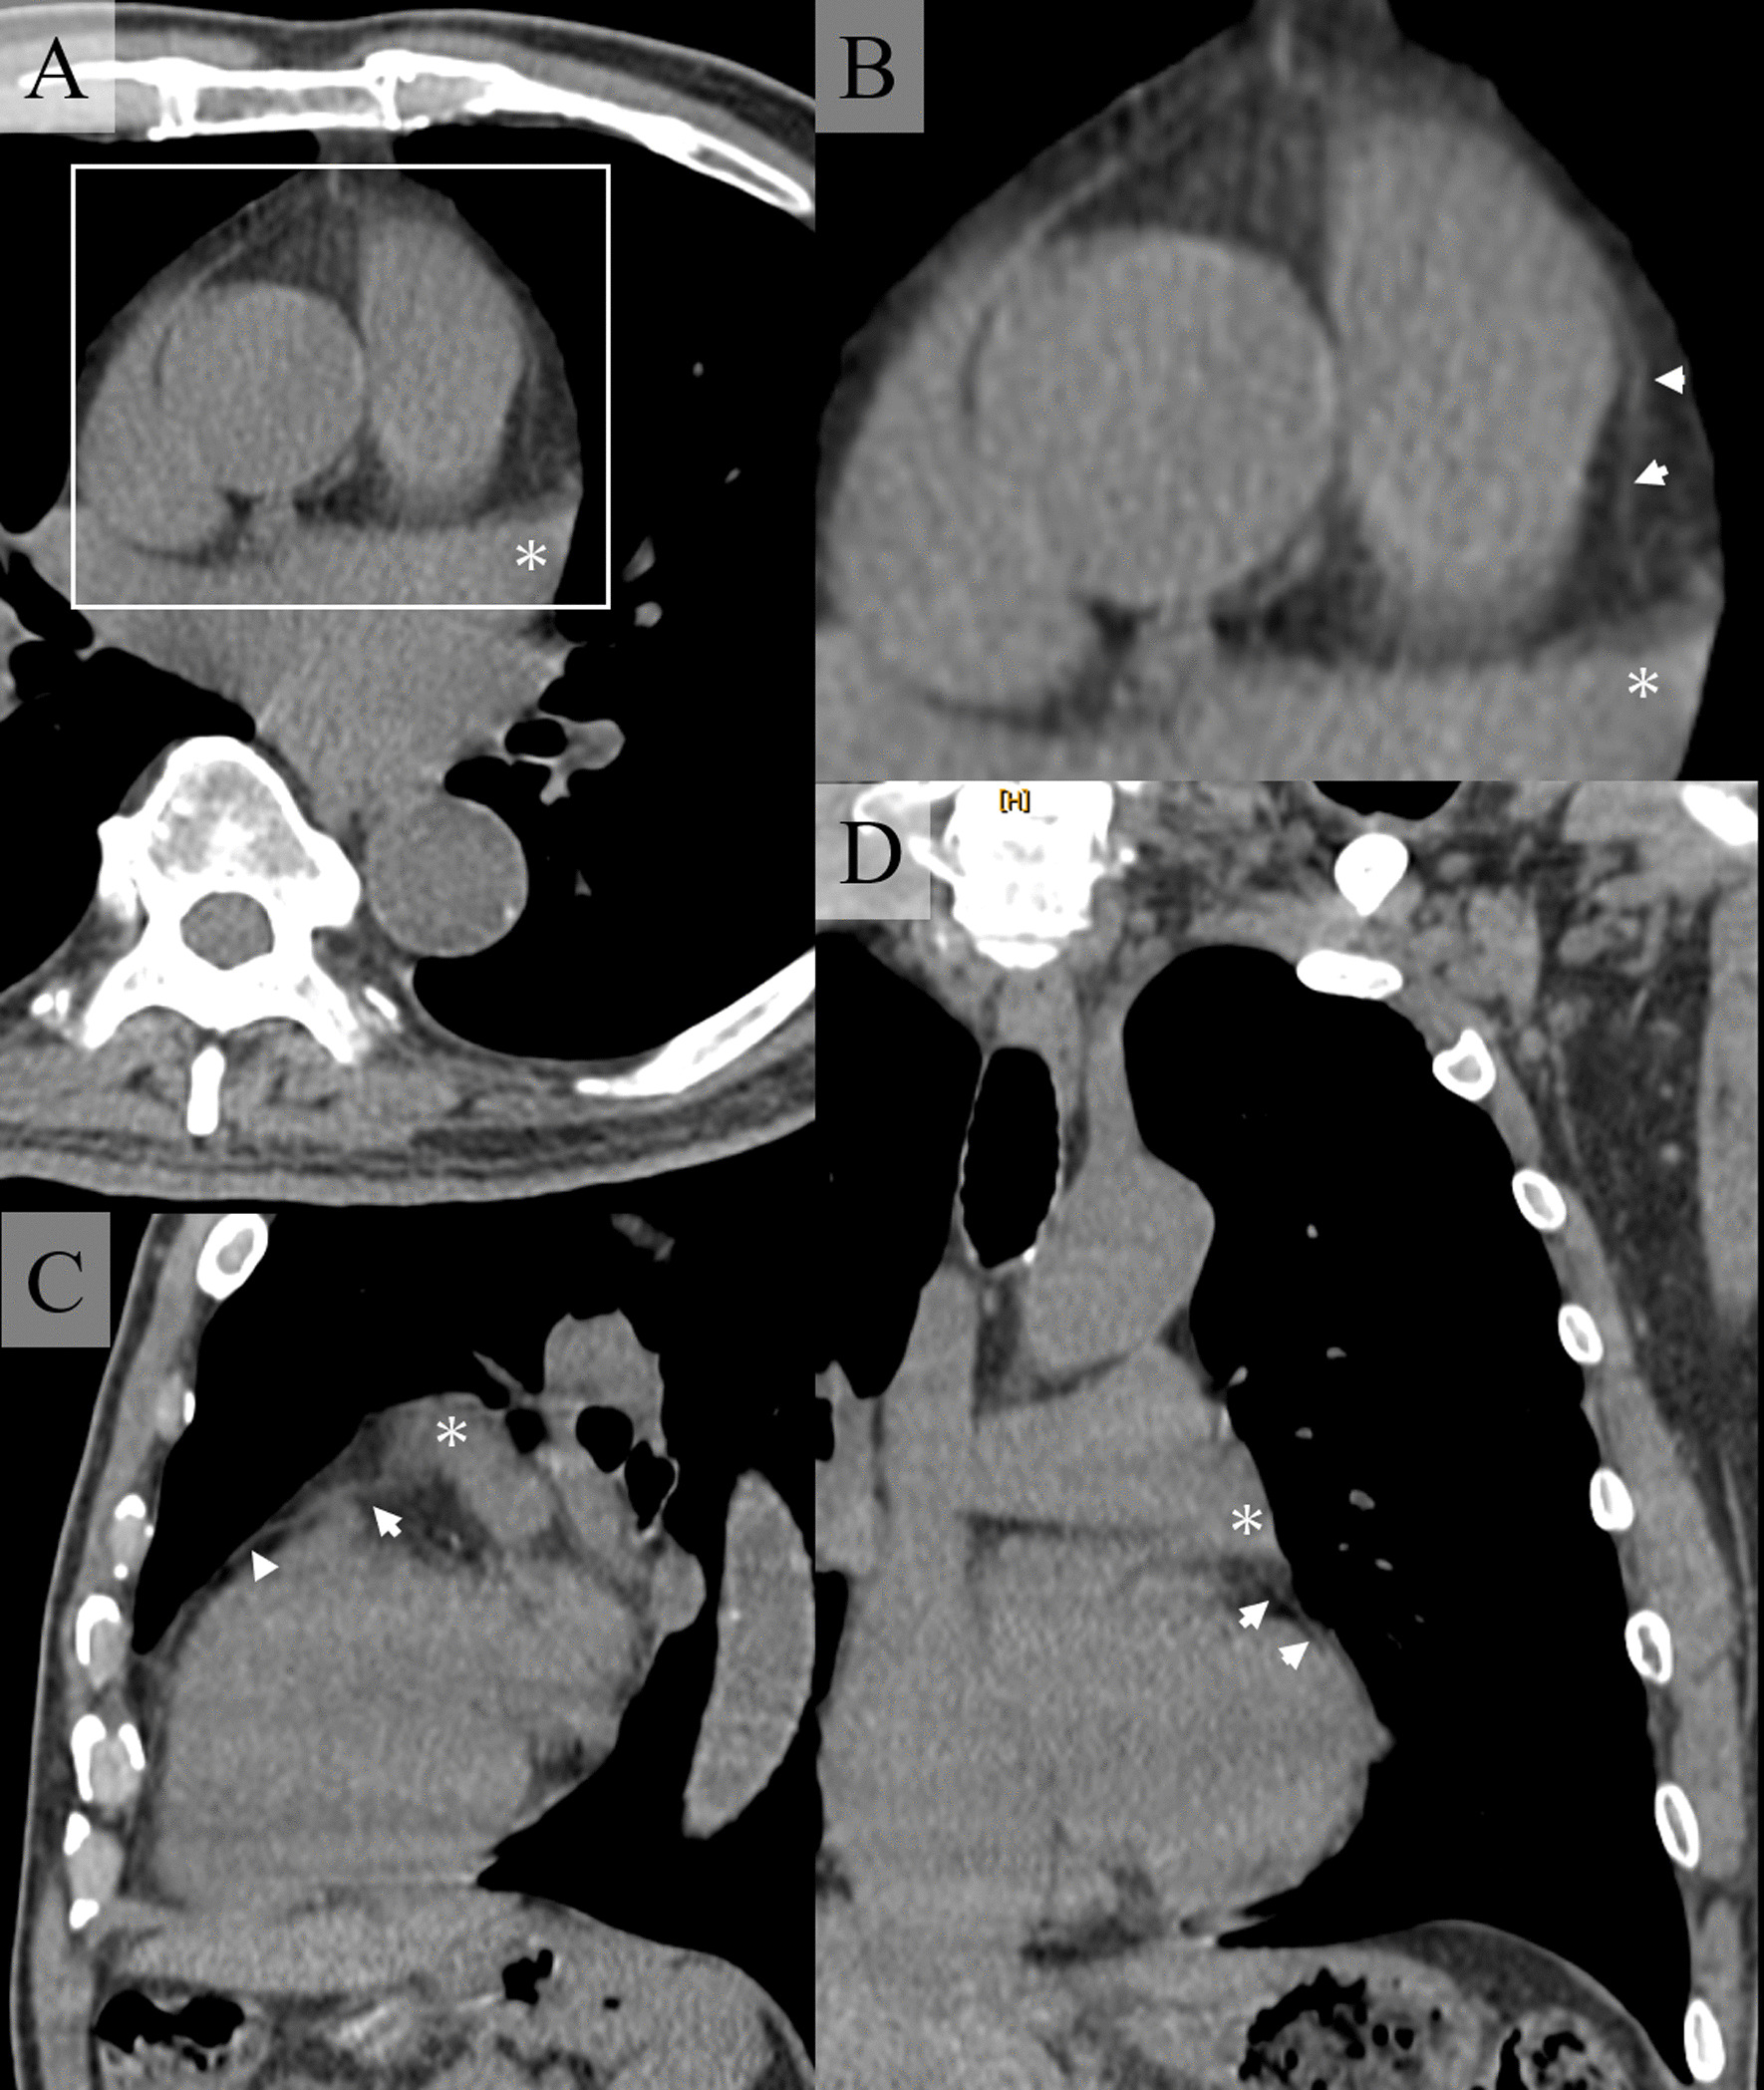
Preoperative CT reconstructed to a thickness of 1 mm. A, B Coronal section, C Sagittal section, D Horizontal section. Asterisk indicates the left atrial appendage. The white arrow head indicates the pericardium. The white square in (A) is the same range as in (B). The pericardium is seen slipping under the left atrial appendage
